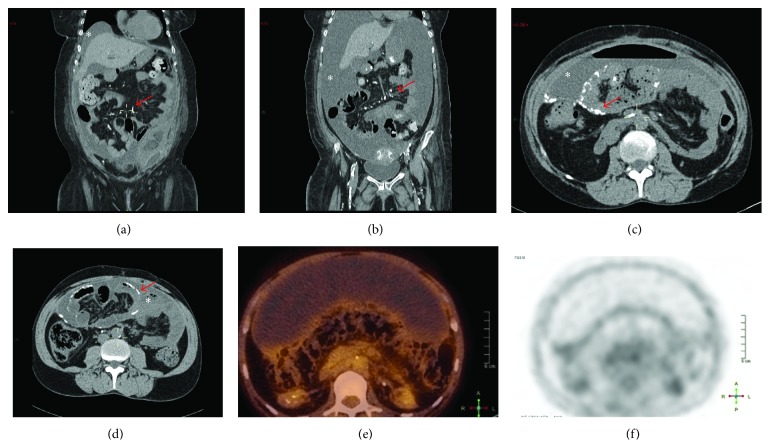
Radiological findings suggesting encapsulating peritoneal sclerosis (EPS). (a, c and b, d correspond to cases EPS 2 and EPS 3, resp.) Abdominal computed tomography without contrast agent showed massive nonloculated fluid and bowel loops drowned into the center of the abdominal cavity suggestive of bowel adhesions. As compared with EPS case  2, EPS case  3 presented more abundant abdominal ascites, marked narrowing of bowel lumen and thickening of visceral and parietal peritoneum, absence of dilated bowel loop, air-fluid levels or entrapped fluid collections, and several calcifications in both parietal and visceral peritoneum. (e and f, case EPS 3) Fluorodeoxyglucose^18^ positron emission tomography (F^18^ PET) scan showed a mild increase in the tracer uptake in the peritoneal areas.
